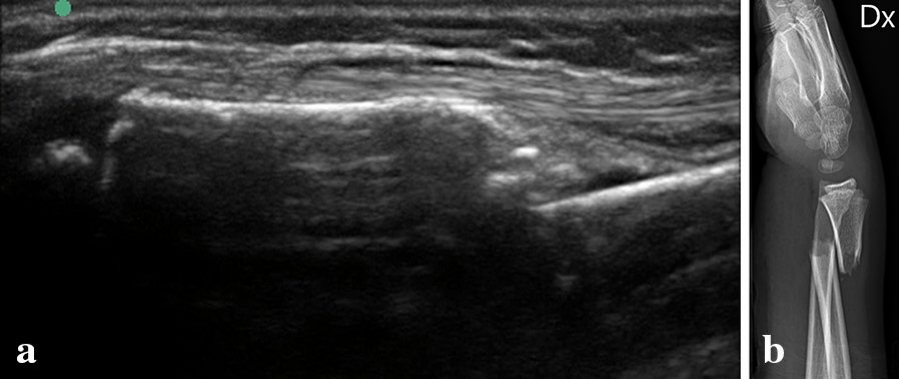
a Displaced complete fracture of the distal radius. US dorsal longitudinal view. b (Same case as image a) Radiograph lateral view. Displaced complete fracture of the distal radius
